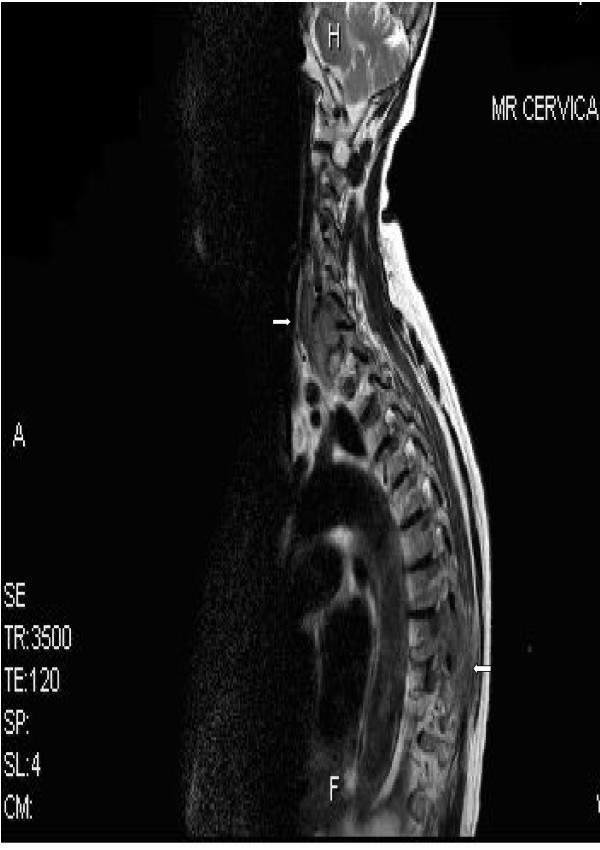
MRI scan shows two soft tissue masses compress the spinal cord at levels T8 and C6/C7.
